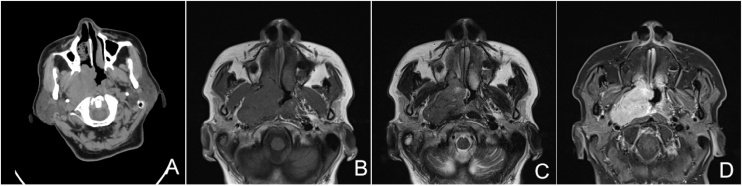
Clinical imaging evaluation of HCCC. Computer Tomography (CT) shows expansive masses with unclear boundaries, infiltrating into surrounding soft and bone tissues on the right posterior wall of the nasopharynx (A). Magnetic Resonance Imaging (MRI) shows a weak T1 signal (B), a strong T2 signal (C), and an obvious enhancement of the fat-suppressed contrast-enhanced T1WI (D).
